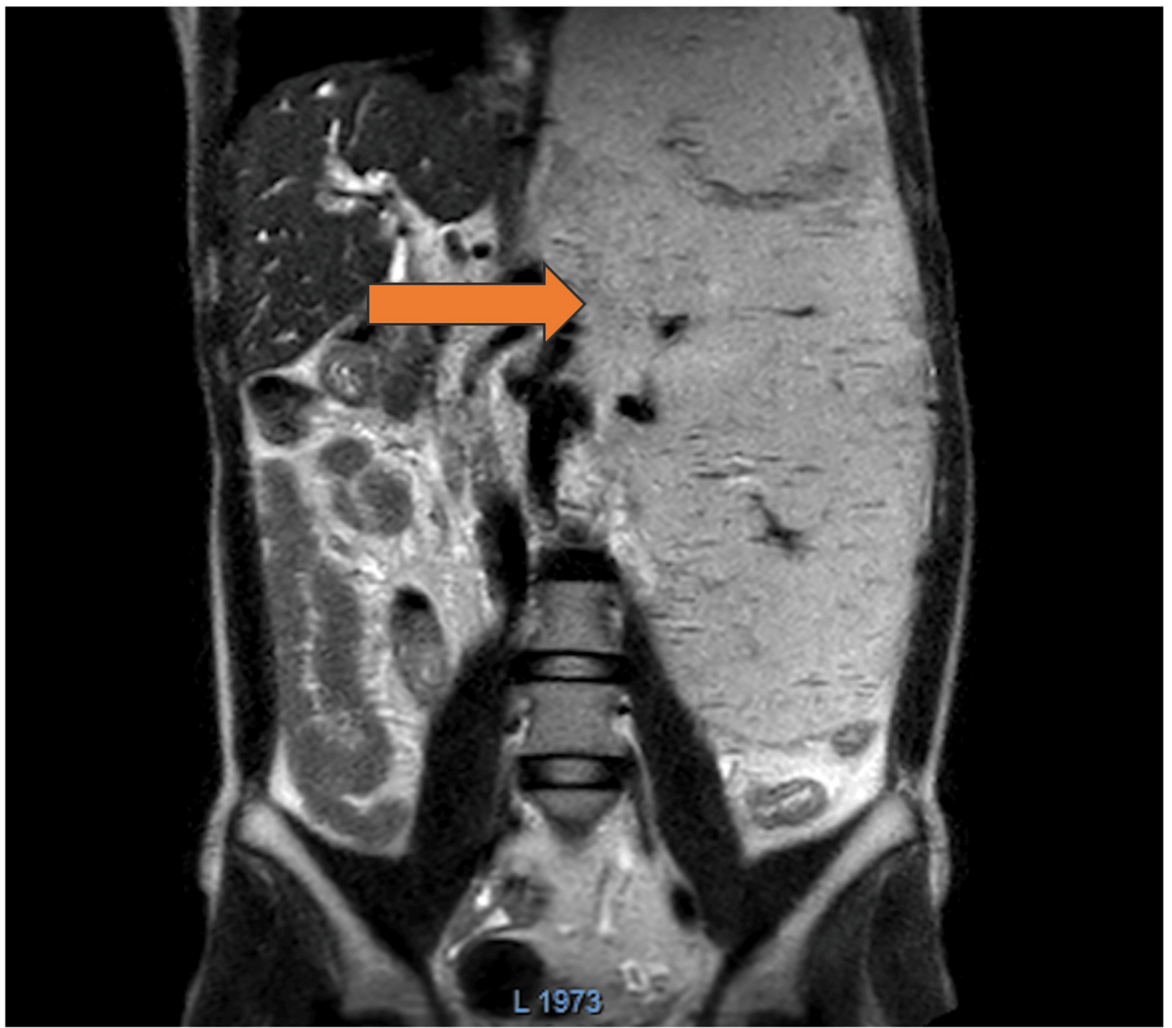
Coronal view of MRI of the abdomen showing massive splenomegaly (arrow mark).
